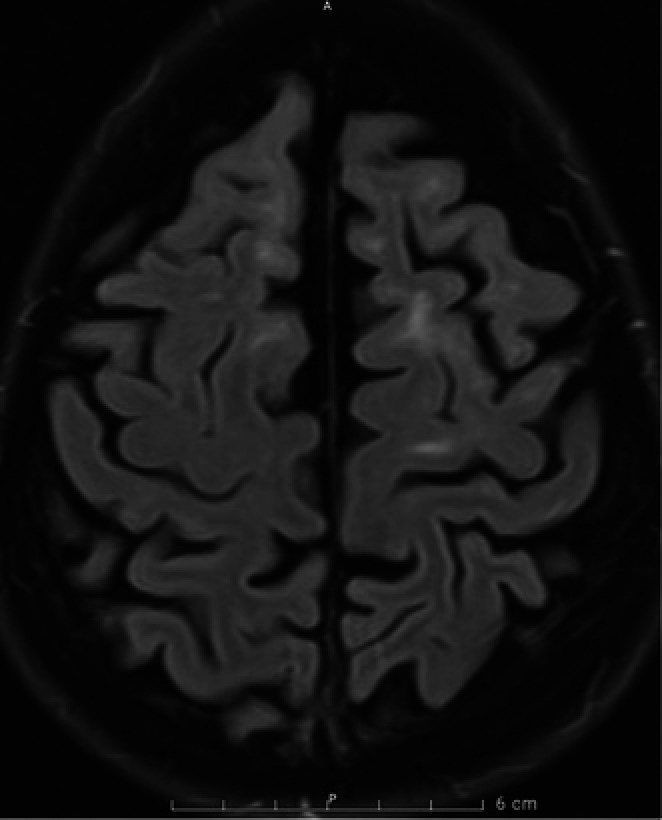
FIGURE 2 4O years‐old. FLAIR. This unusual pattern raised the hypothesis of a leukodystrophy.
